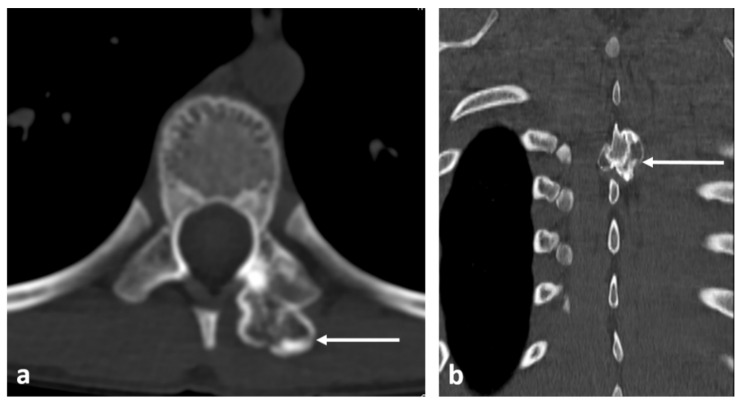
Axial (a) and coronal (b) CT images demonstrating a pedunculated osteochondroma of a thoracic vertebra (white arrows). Well defined lesion with continuity with cortex and medulla are typical features.
